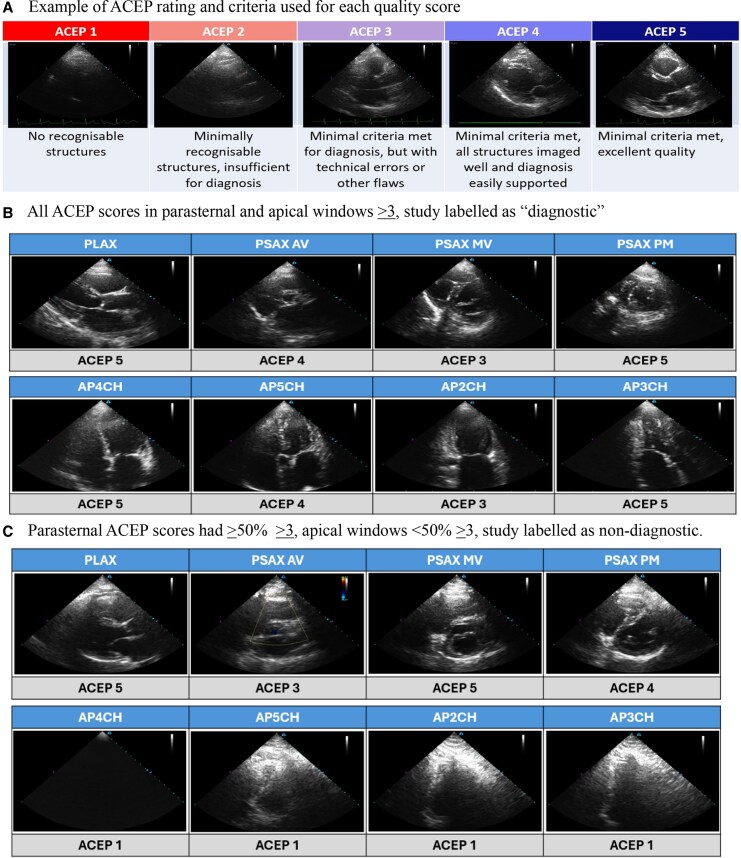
Categorization of image-quality AI-POCUS scans and example cases of diagnostic vs. non-diagnostic quality. (*A*) Example of ACEP rating and criteria used for each quality score. (*B*) All ACEP scores in parasternal and apical windows >3, study labelled as ‘diagnostic’. (*C*) Parasternal ACEP scores had ≥50% ≥ 3, apical windows <50% ≥ 3, study labelled as non-diagnostic.
